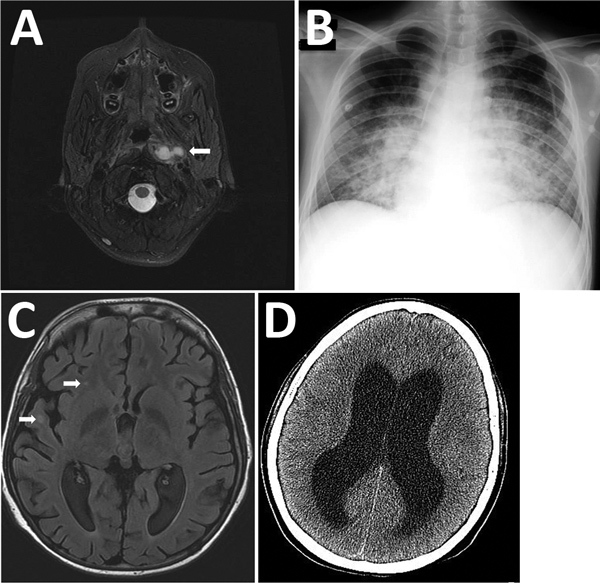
Most relevant imaging results from a 16-year-old boy with X-linked chronic granulomatous disease who died of meningitis caused by a virulent *Granulibacter bethesdensis* strain. A) Computed tomography image showing deep cervical abscess (arrow), July 2012. B) Radiograph showing pneumonia with pleural effusion, October 2012. C) Cranial magnetic resonance image showing multiple intraparenchymal brain abscesses (arrows), December 2012. D) The patient died of obstructive hydrocephalus (shown) and multiorgan failure, April 2013.
